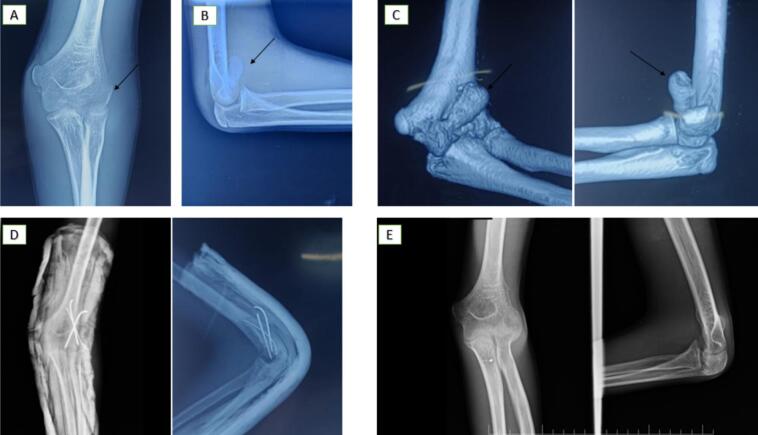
Case 2: Anterior Capitellar Shear Fracture (Type IV - McKee) with Proximal and Medial Displacement. (A) Anteroposterior plain radiograph showing a lateral fracture fragment initially missed (black arrows). (B) Lateral X-ray revealing a double contour sign. (C) 3D CT scan confirming the anterior capitellar shear fracture (Type IV - McKee) with proximal and medial displacement (black arrows). (D) Postoperative anteroposterior and lateral radiographs showing proper reduction and K-wire placement. (E) Follow-up radiographs at 6 months post-surgery confirming fracture union and anatomical alignment.
